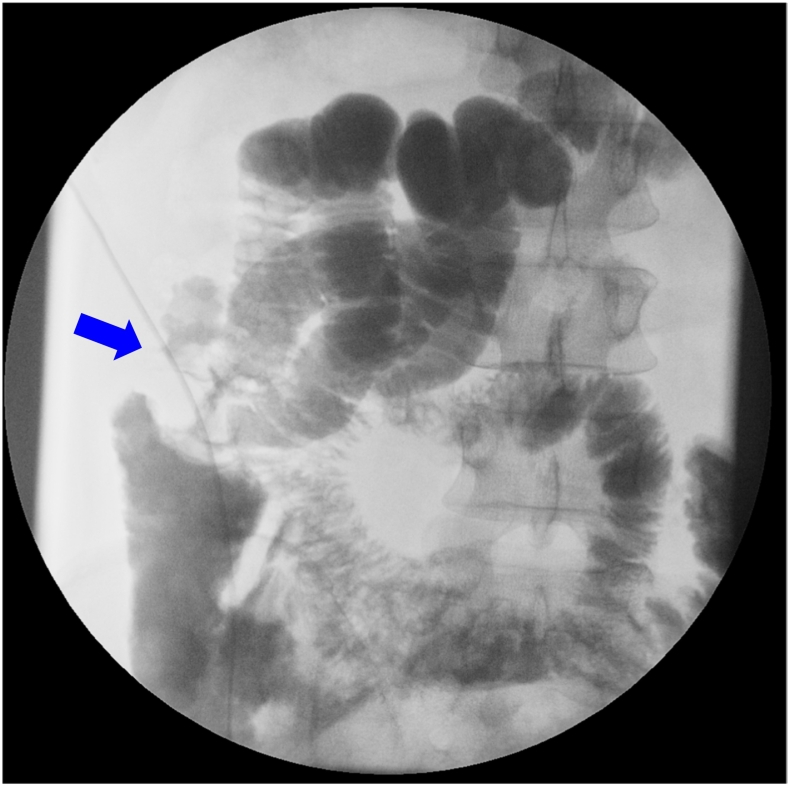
A radiographic contrast enema performed after the first surgery. A long radiopaque transnasal tube was inserted. Stenosis still remained (blue arrow), but fluid easily passed through the lesion. (For interpretation of the references to colour in this figure legend, the reader is referred to the web version of this article.)
